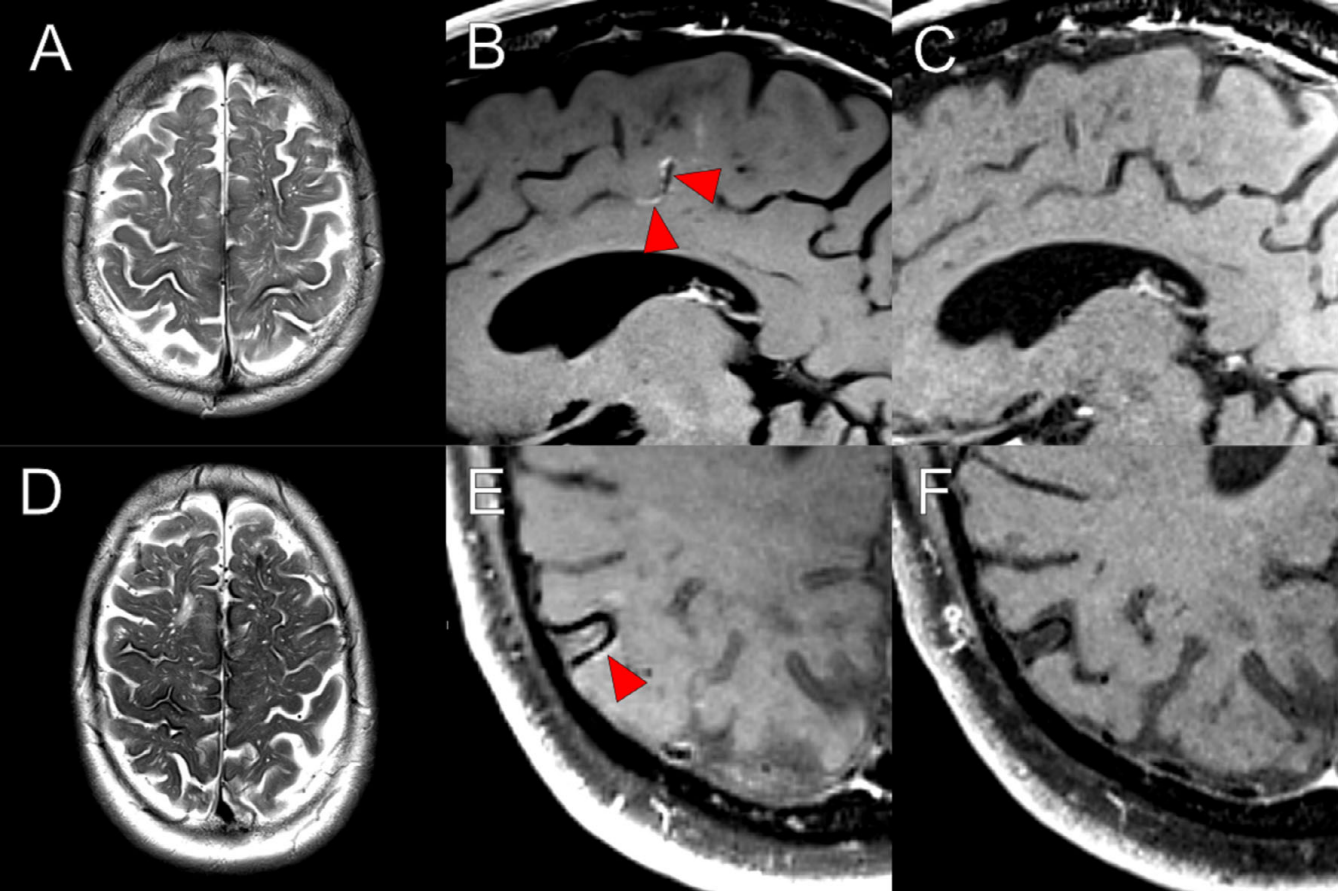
Each row contains magnetic resonance imaging for cases 5 and 6. In case 5, there was extensive superficial siderosis and many enlarged subcortical perivascular spaces (A). Vessel wall imaging showed irregular mural enhancement in a distal branch of the left anterior cerebral artery (*red arrows*, B), as well as a wider region of leptomeningeal enhancement, which resolved on follow‐up imaging 5 months later (C). In case 6, there was also extensive superficial siderosis and many enlarged perivascular spaces (D). A distal right middle cerebral artery branch showed a short segment of mural enhancement (E, *red arrow*) that resolved on follow‐up imaging 4 months later (F). [Color figure can be viewed at www.annalsofneurology.org]
